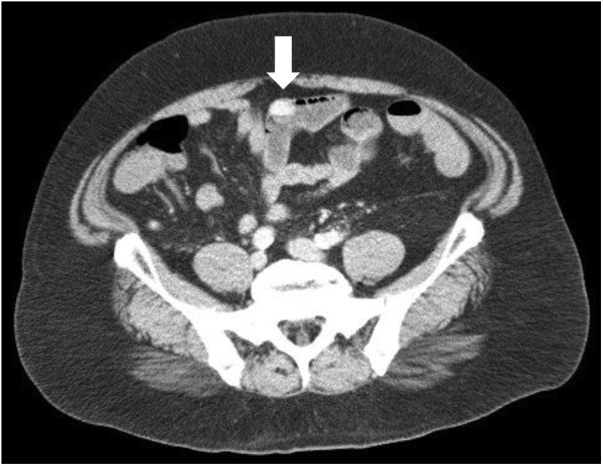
Contrast-enhanced CT shows a hyper-vascularized tumor in the small bowel (white arrow).
